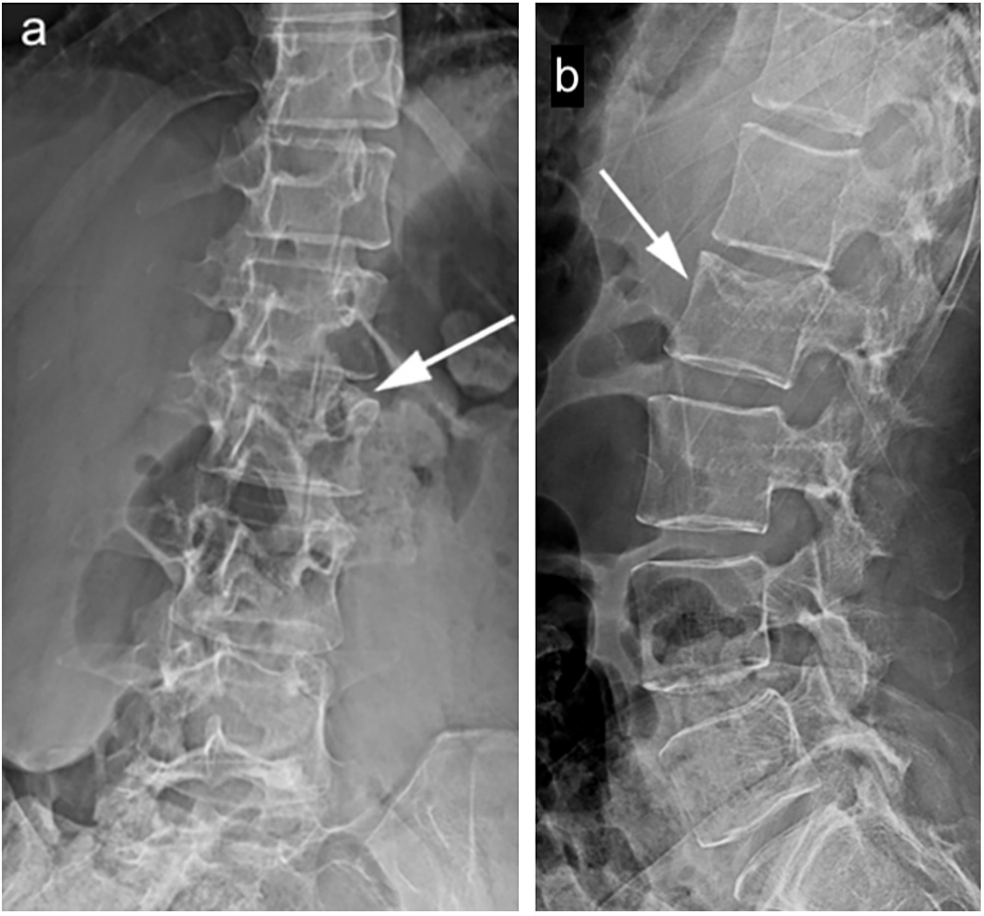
(a) Anteroposterior and (b) lateral radiographic follow-up 11 months after hardware removal in the same patient as Figures 6a, 6b showing no increased kyphosis.
